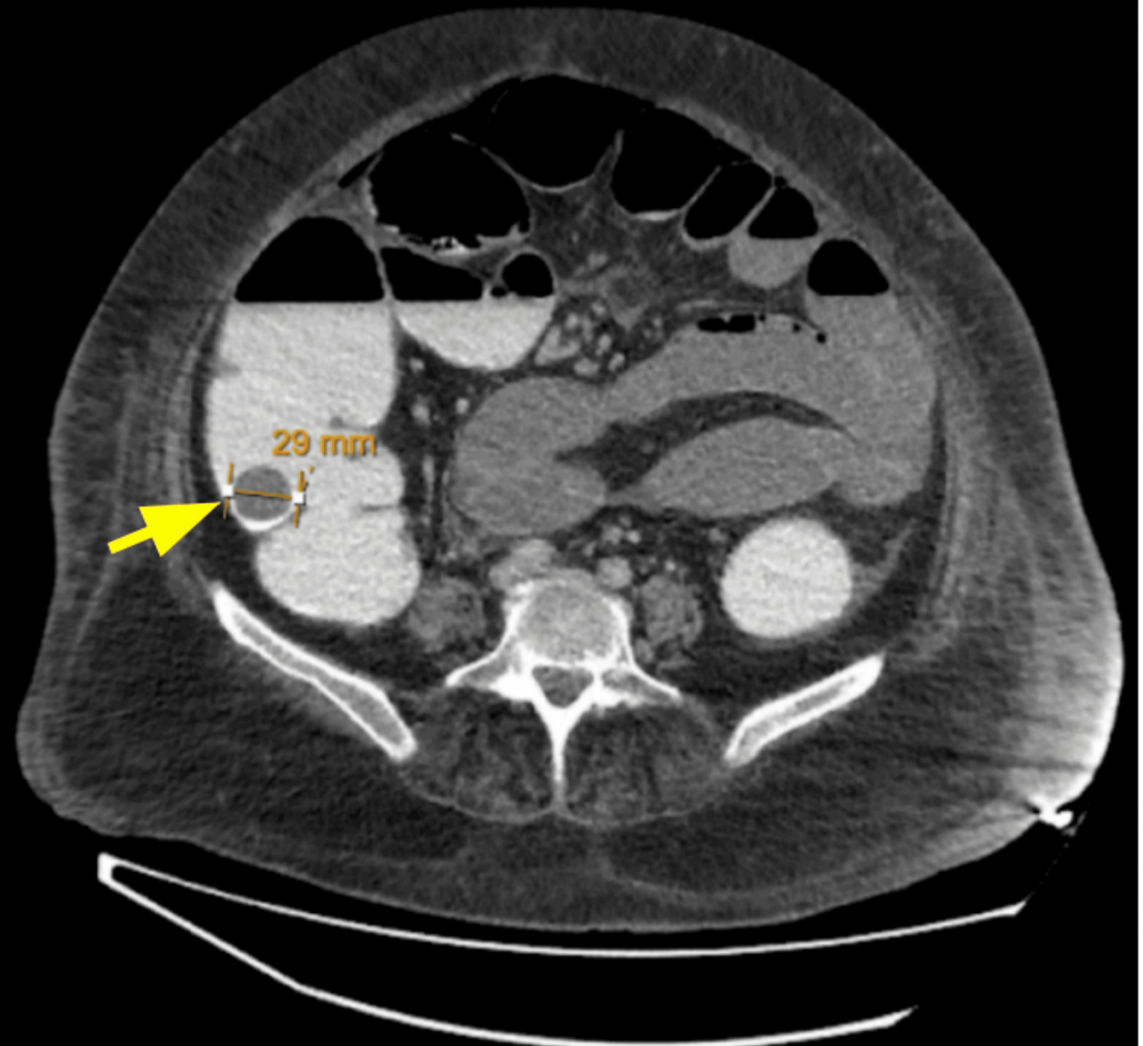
Axial CT image of the abdomen showing a 29 mm gallstone in the cecum, indicated by a yellow arrow
